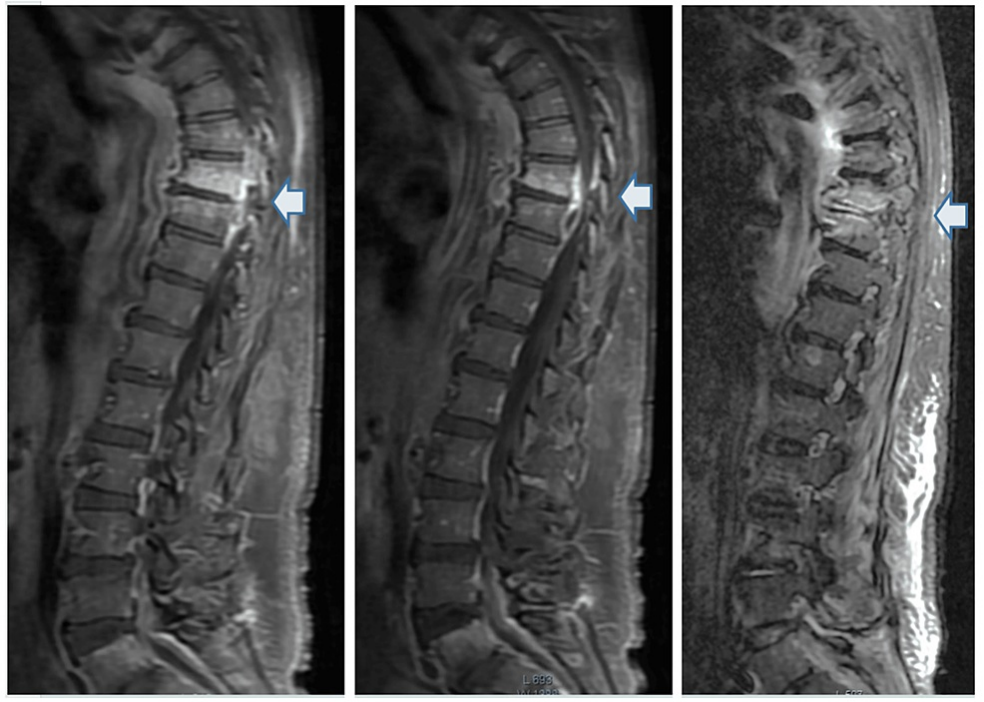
Pott's disease Magnetic resonance imaging (MRI) of the spine (sagittal view) showing exuberant dorsolumbar spondylodiscitis and epidural empyema with mass effect on the spinal cord (arrows).
